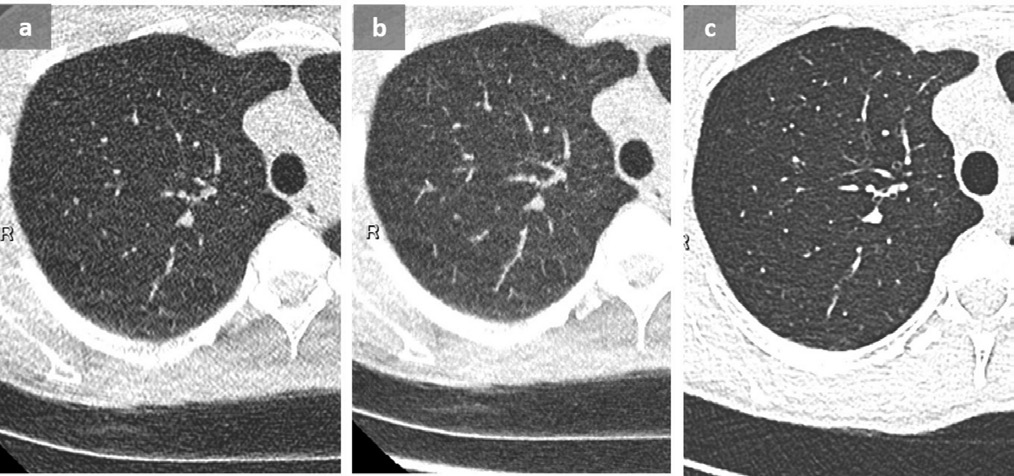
When performing an acquisition with a very low CTDI at 0.11 mGy, image noise, well seen outside of the chest, can mimic a miliary disease on thin axial slice (a) and MIP reformat (b), even though applying an iterative reconstruction algorithm. A follow-up CT with a CTDI at 0.29 mGy (b) reduces noise, allowing to exclude micronodules. These CT were performed in a context of recurrent pneumothorax in a young patient with endometriosis (not shown). CTDI, CT dose index; MIP, maximum intensity projection.
